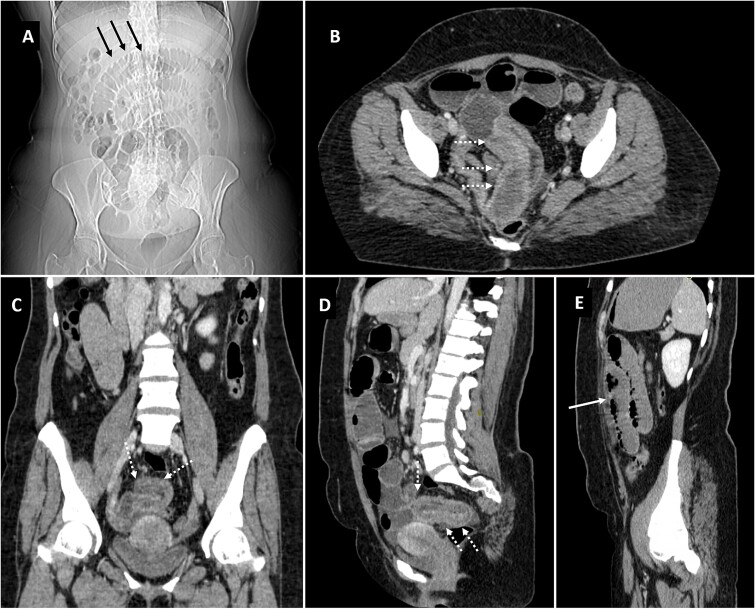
Scannogram (A) showing dilated small bowel loops in central abdomen (black arrows). Axial (B), coronal (C) and sagittal (D, E) images of contrast CT showing telescoping of pelvic ileal loop (dotted white arrows) with upstream small bowel obstruction (solid white arrow).
